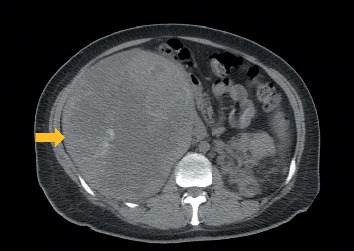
CT abdomen without contrast showing a large, right suprarenal vs. adrenal mass (arrow).
